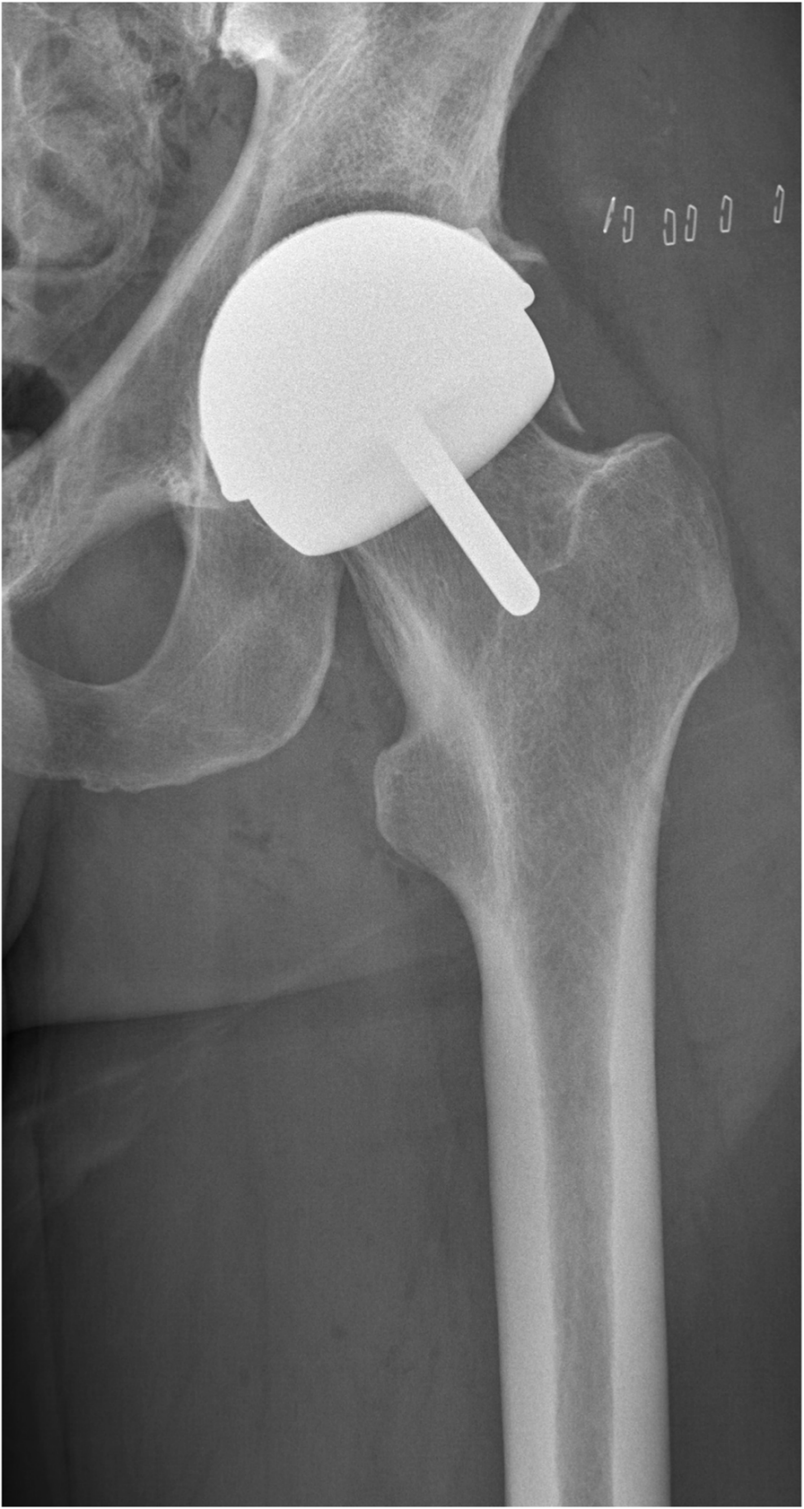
Postoperative x-ray (MoM-HR)
